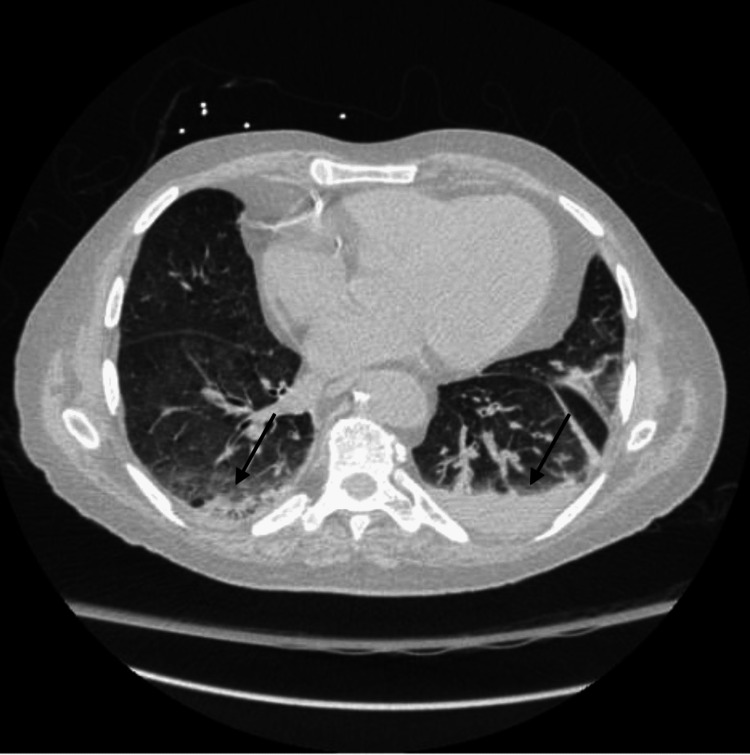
Chest CT scan Chest CT scan demonstrates bilateral pleural effusions and pulmonary infiltrates, as indicated by the arrows.
